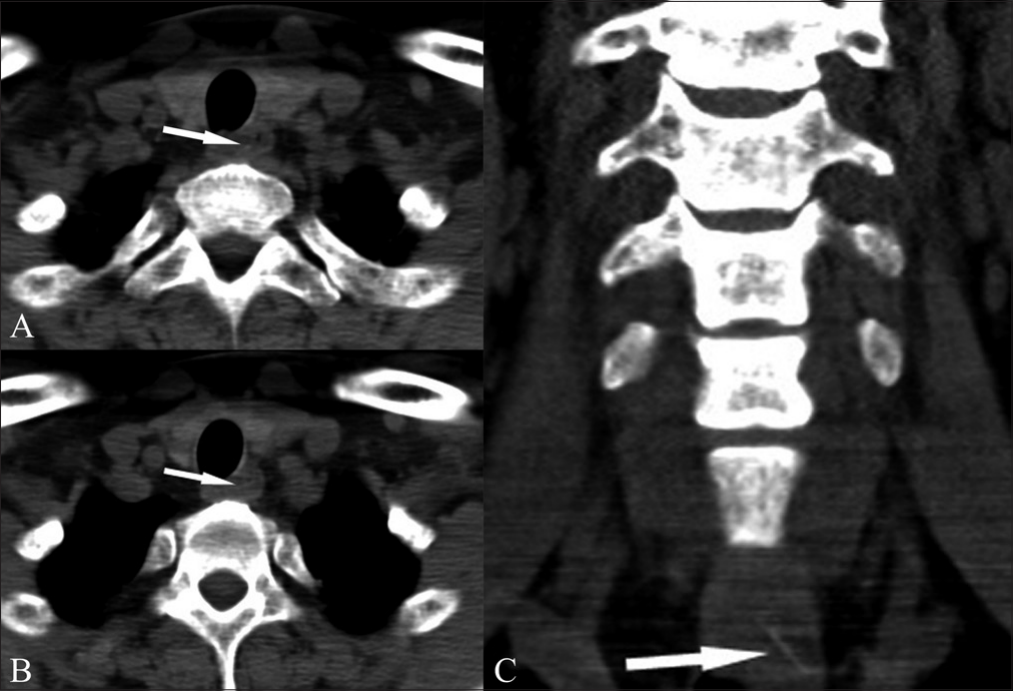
Non-contrast axial (A,B) CT scans show a questionable hyperdensity within the esophageal lumen, at the level of the thoracic inlet (arrows). The coronal reconstructed image however clearly shows the presence of a fine linear FB (arrow in C). Coronal images are in general more useful in cases of FB since the bone is usually oriented orthogonally to the acquired axial images
